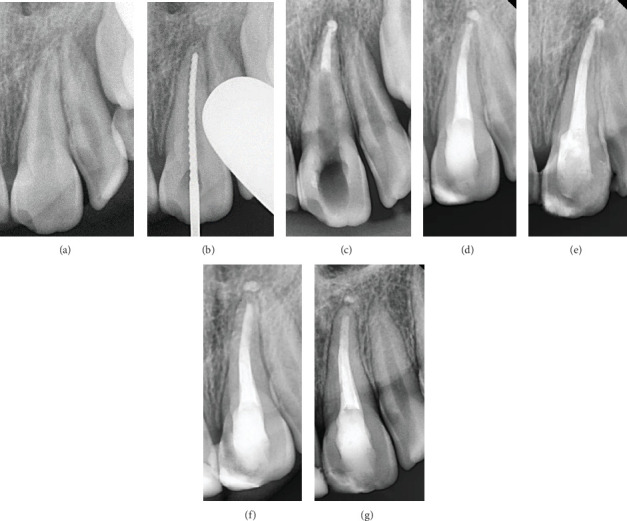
Case 1. (a) Preoperative periapical radiograph. (b) Working length radiograph. (c) Intermediate radiograph showing apical plug formation. (d) Postoperative radiograph. (e) A 6-month recall radiograph. (f) A 12-month recall radiograph. (g) A 24-month recall radiograph.
